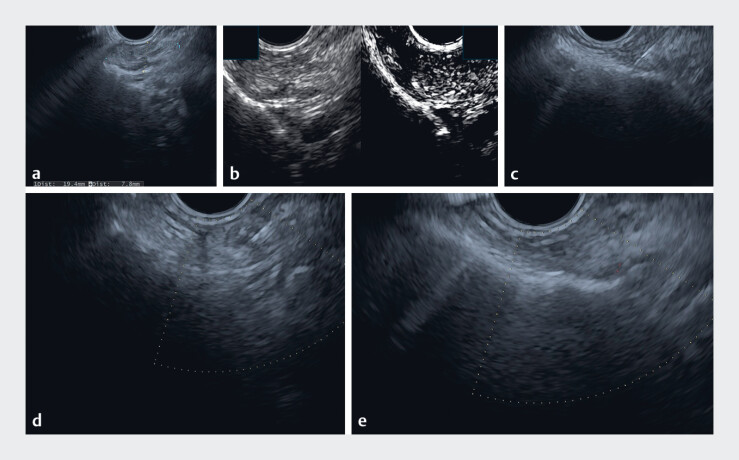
Endoscopic ultrasound (EUS) images showing the stages of the EUS-guided fine-needle
